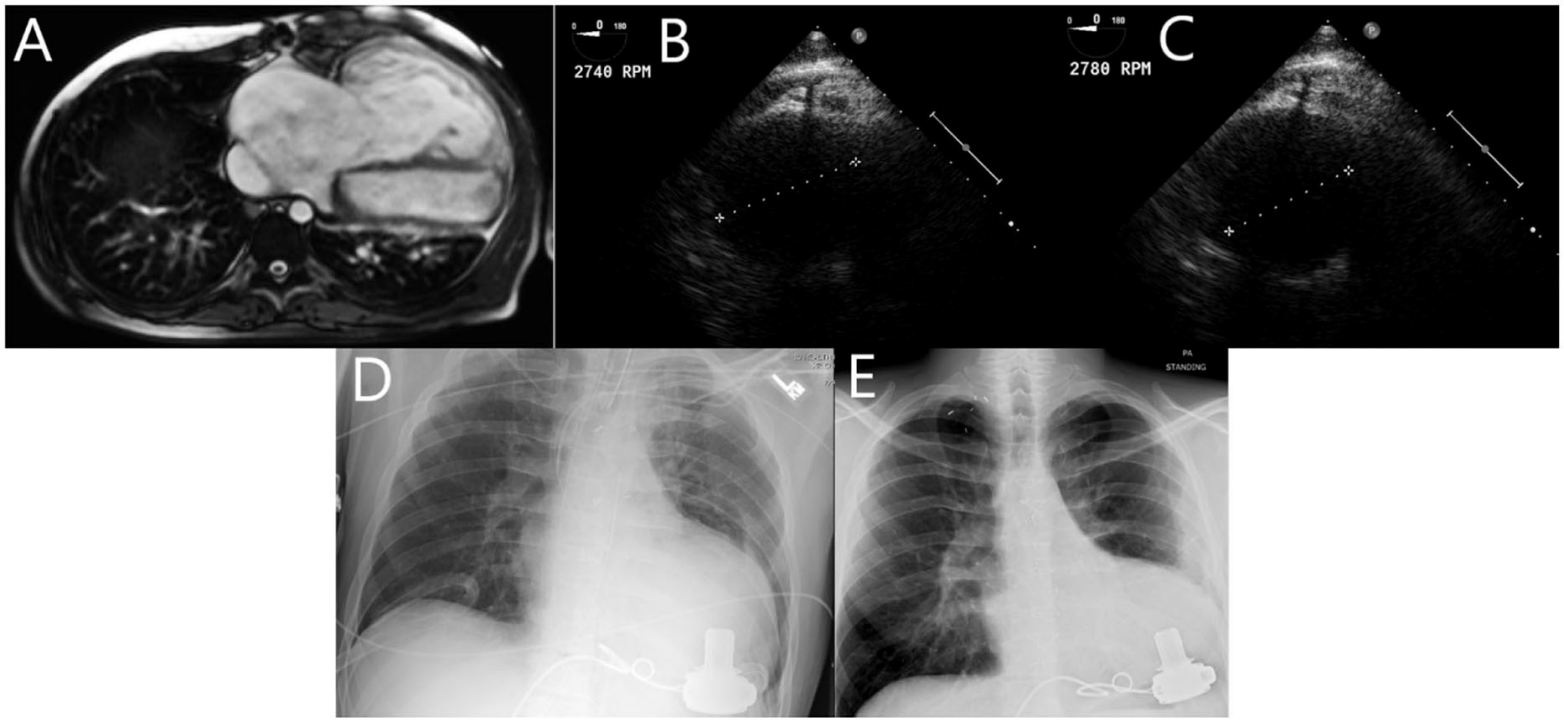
(A): Cardiac MRI showing severely dilated and hypertrophied RV with mildly diminished systolic function. (B, C): TEE monitoring showing RV chamber size decrease from 6.2 cm at low VAD speed (2,740 RPM) to 5.3 cm at high VAD speed (2,780 RPM) (D, E): Chest x-ray showing significant decrease in RV size between post-operative day 1 and 10 months post-operatively.
